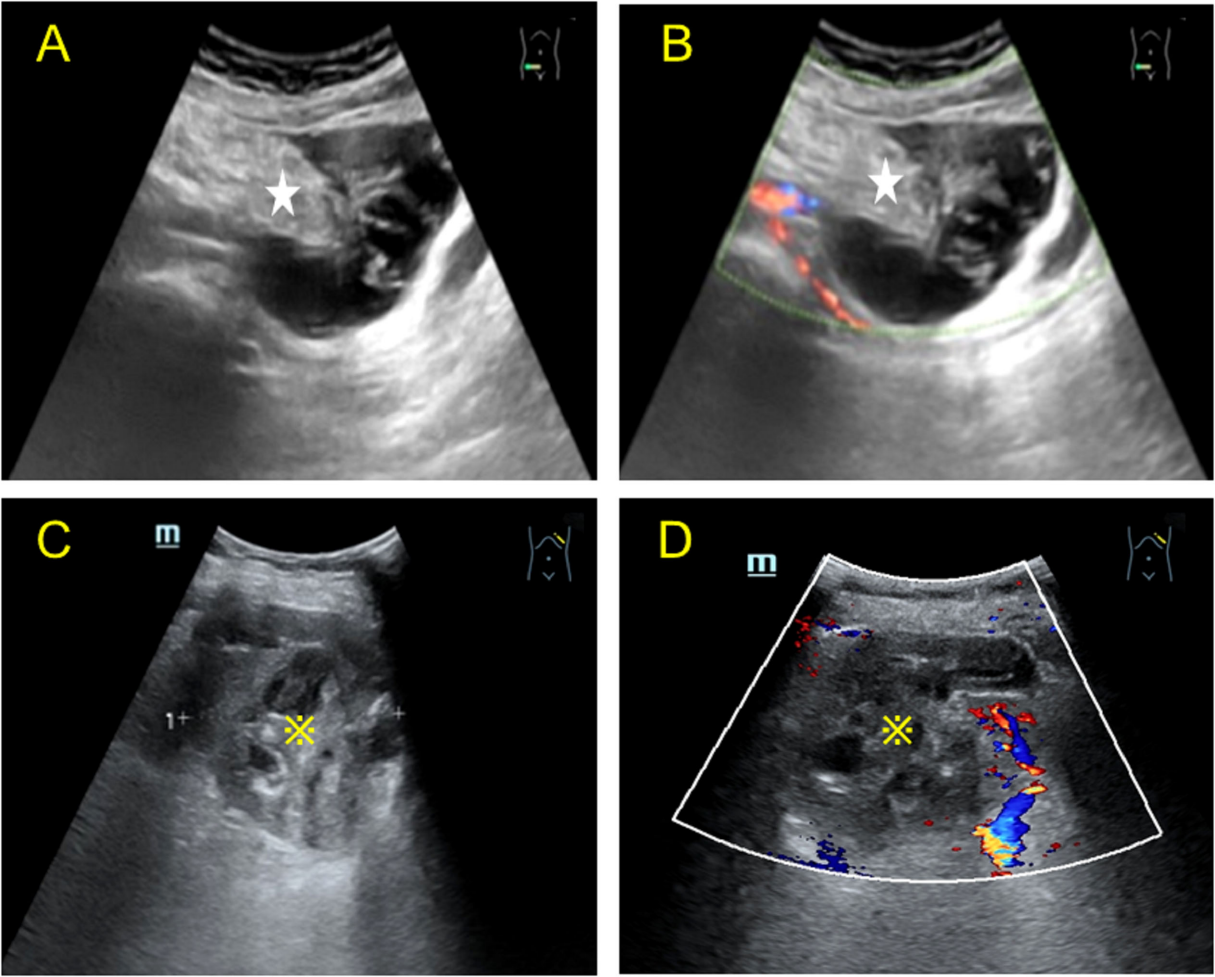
Two-dimensional Color Doppler images of the lesion in the right lower abdomen and spleen obtained by transabdominal ultrasound. (A) Longitudinal view of the transabdominal ultrasound scan of the right lower abdomen, showing a mixed cystic and solid echo. The solid part (asterisk) was eccentric, most of the surrounding tissue was cystic, and a radial stripe light band was seen in the dark area connected to the solid part. (B) Color Doppler of the mass showed minimal linear blood flow in the solid part; heterogeneous echogenicity was observed in the right adnexa, mainly anechoic around some hyperechoic echogenicity in the center. (C) Transabdominal ultrasound of the spleen showing a mixed cystic and solid mass (※) in the left hypochondrium, with irregular dark liquefied areas septate (left-right diameter of the mass: 7.52 cm). (D) Color Doppler image showing sparse blood flow in the solid part and no significant blood flow distribution in the cystic part.
